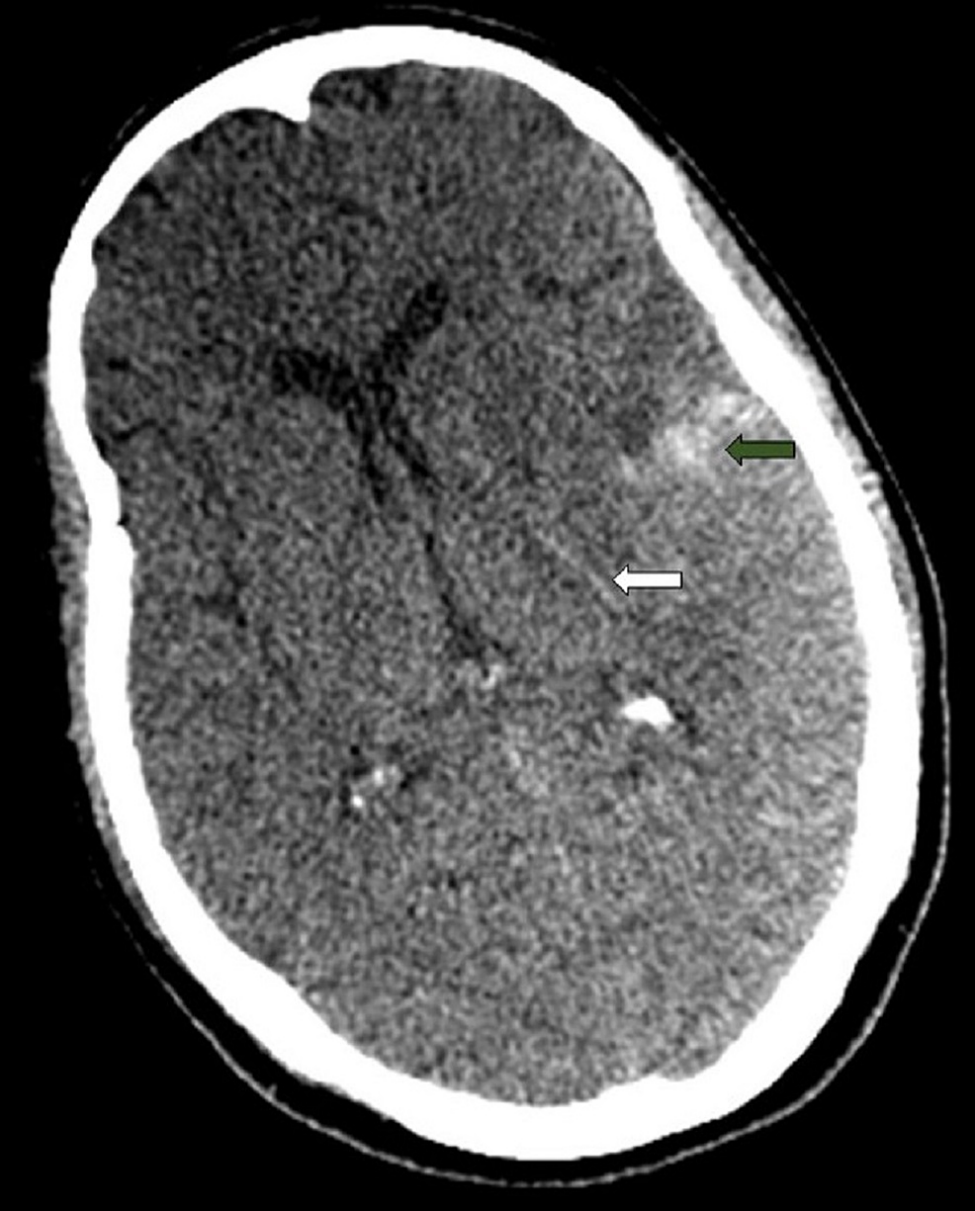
Cerebral CT, axial section, without injection of contrast. Subarachnoid meningeal hemorrhage (white arrow) and intraparenchymal hematoma (green arrow). CT: computed tomography
